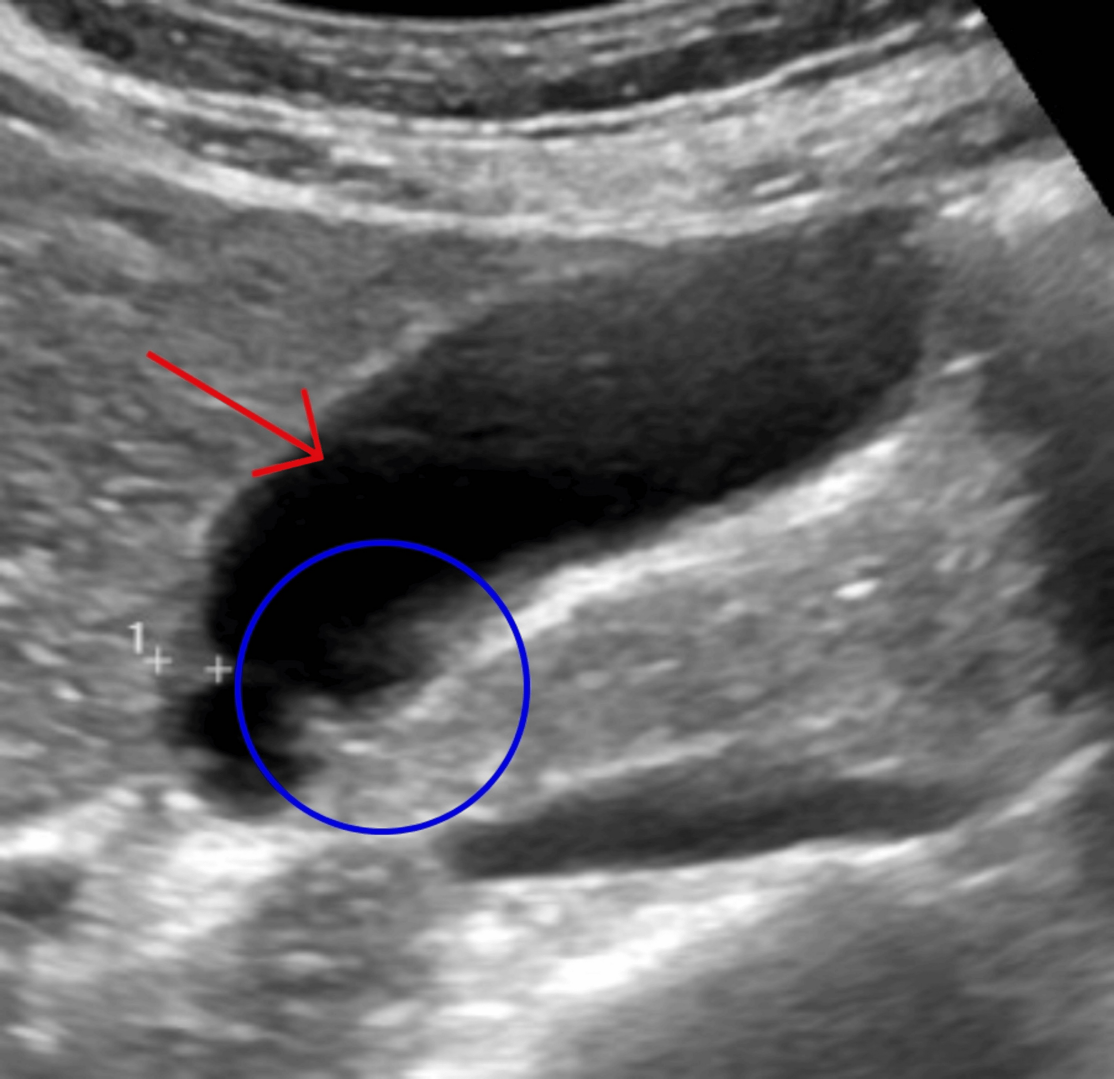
Ultrasonography of the gallbladder showing 2-3 polyps, no other abnormalities noted. Red arrow pointing to the gallbladder. Blue circle showing the gallbladder polyps.
